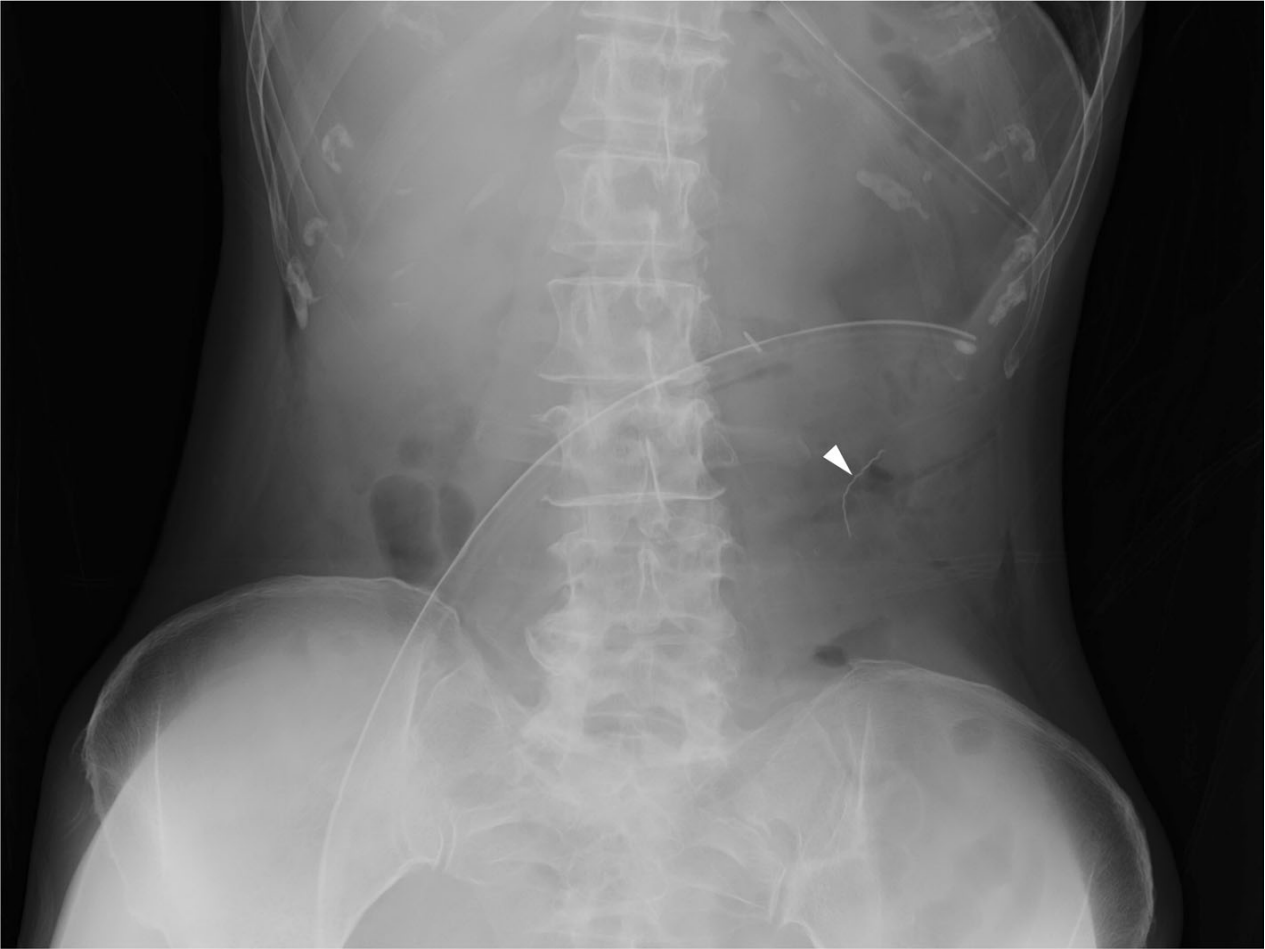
An X-ray image of the abdomen showed a radiopaque thread-like substance retained in the left part of the abdomen (arrowhead)
